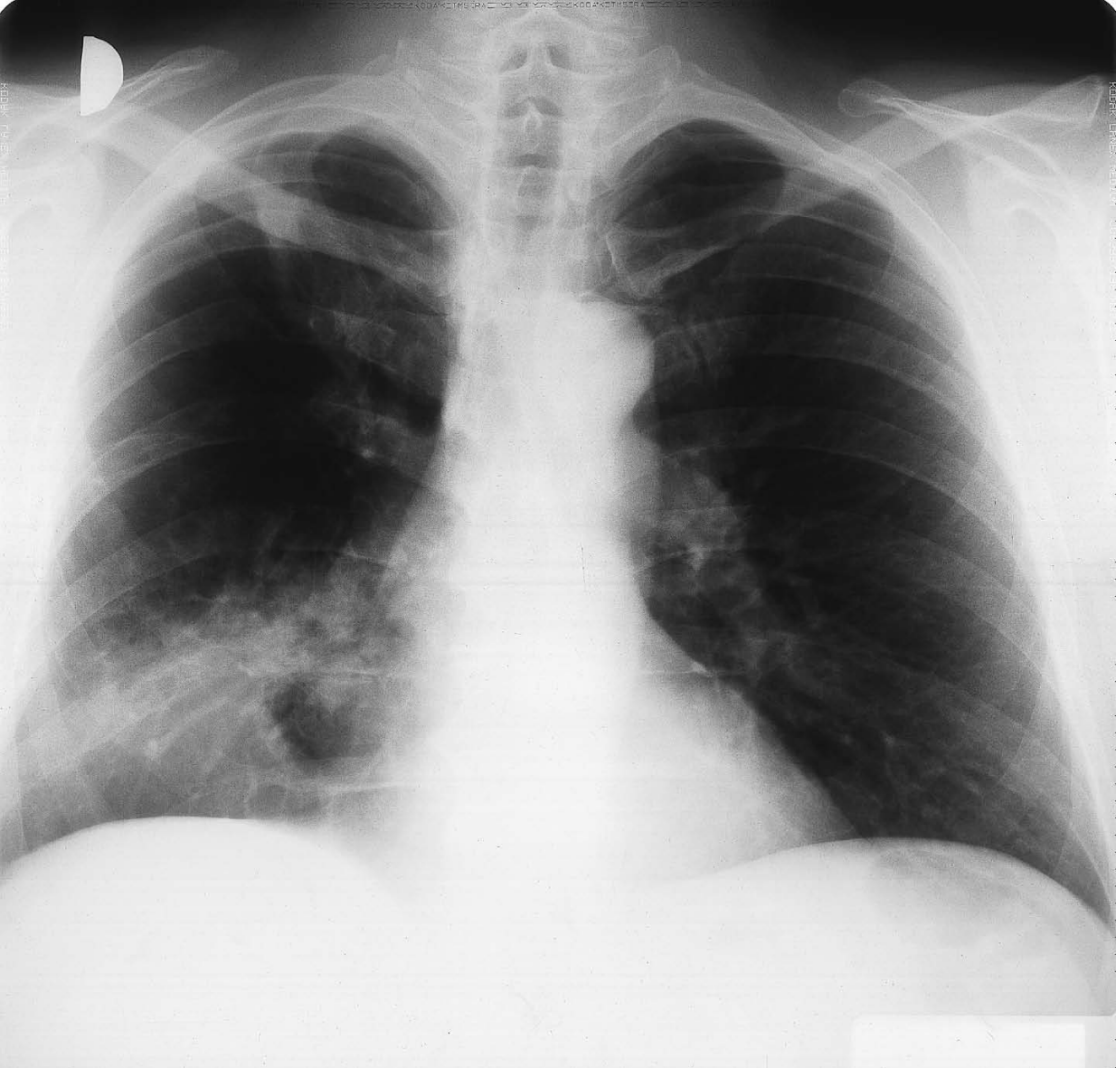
Chest radiograph showing an infiltrate with a cavitation in the right lower and middle lobes.
